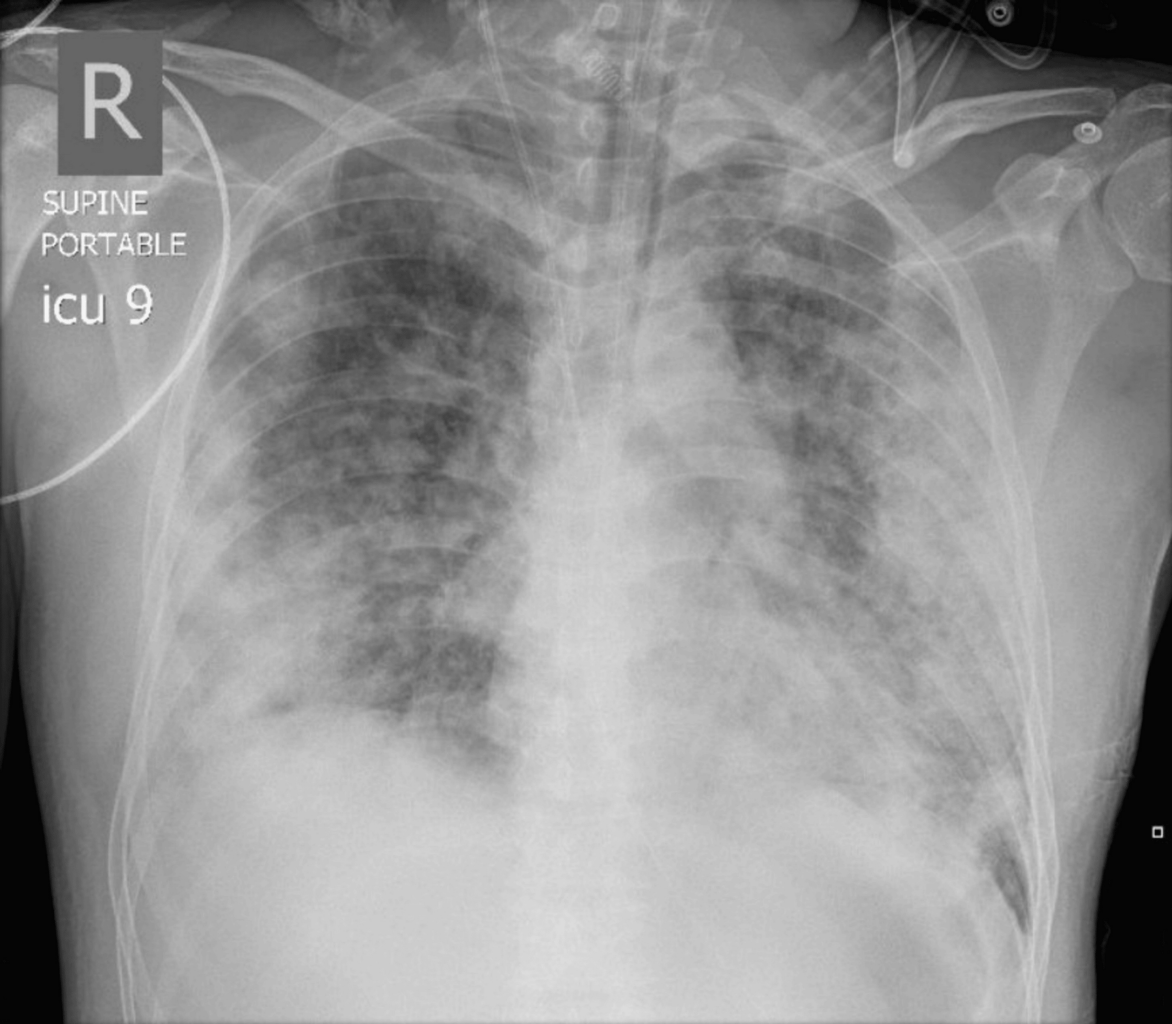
Chest X-ray showing multiple cavities
